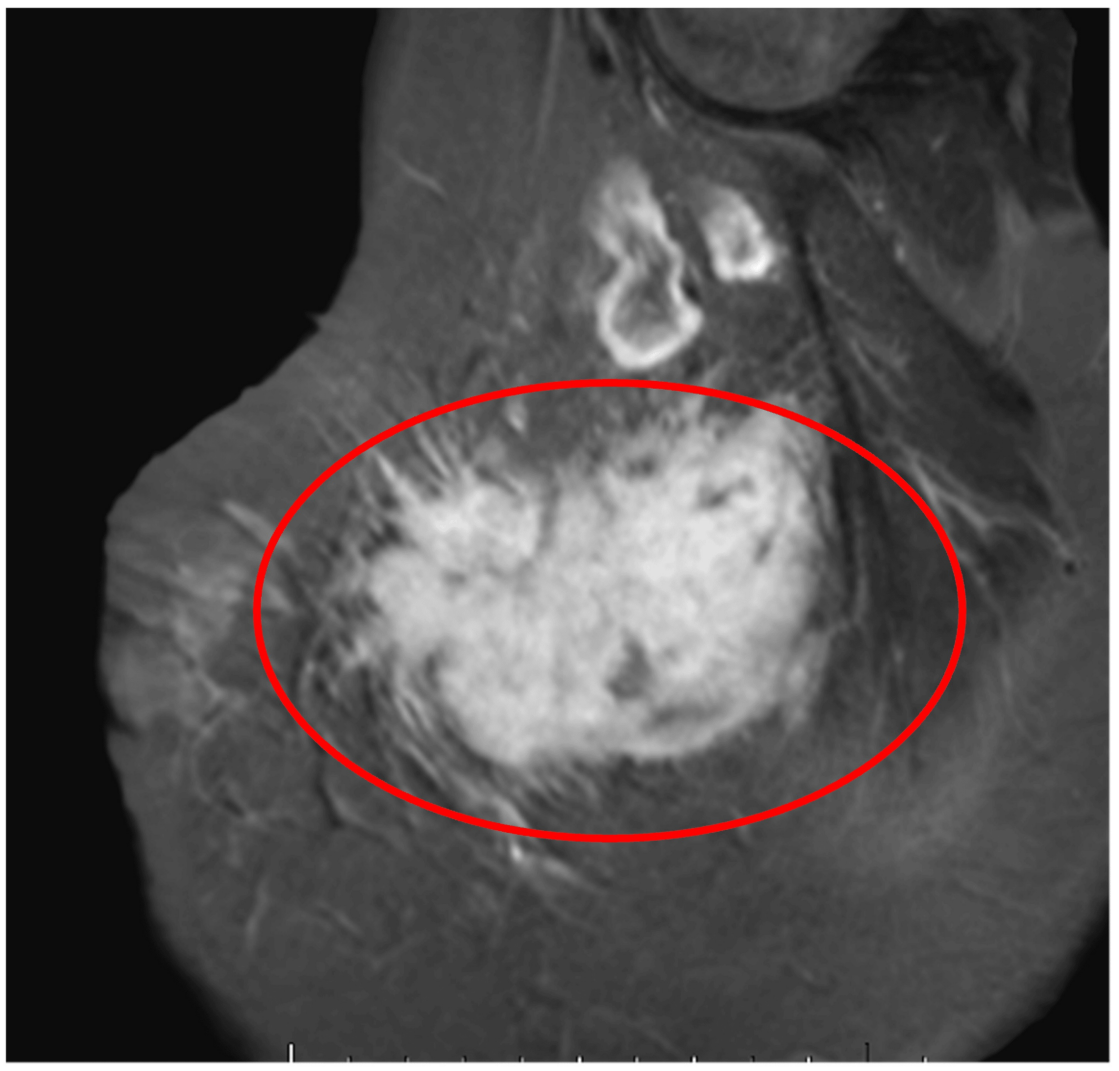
Sagittal T2-weighted STIR scan of the left breast Sagittal T2-weighted STIR scan of the left breast (repetition time scan/echo time msec, 3.5/57, section thickness 5mm) showed the mass to be hyperintense. STIR: Short Tau Inversion Recovery
